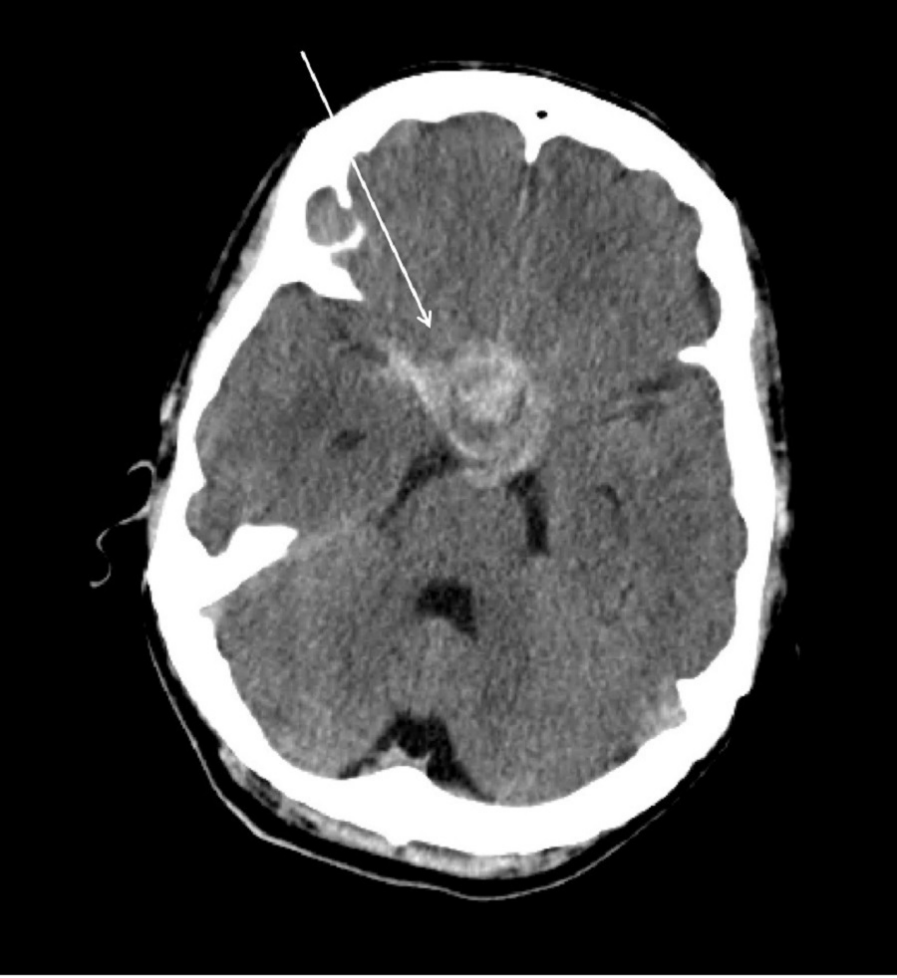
Preoperative head computed tomography (CT) without contrast demonstrating sellar mass extending into the left cavernous sinus.
